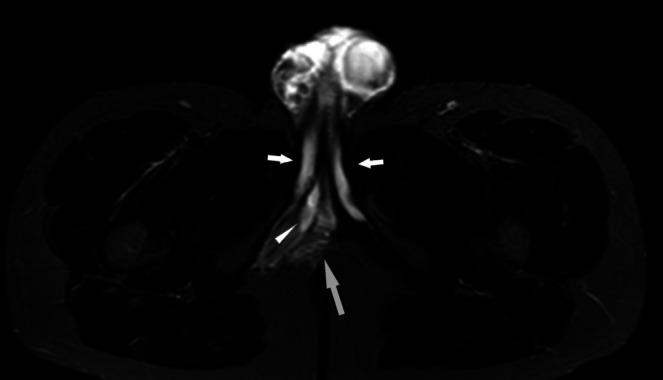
Axial STIR sequence MRI image of the perineum including the base of the penis. The two white tubular structures on each side are the corpora cavernosa (white arrows). The middle structure, which lies on the right side of the midline is the haematoma (white arrowhead), which together with the oedema (grey arrow) implies injury to the right corpus cavernosum, not shown on this image.
